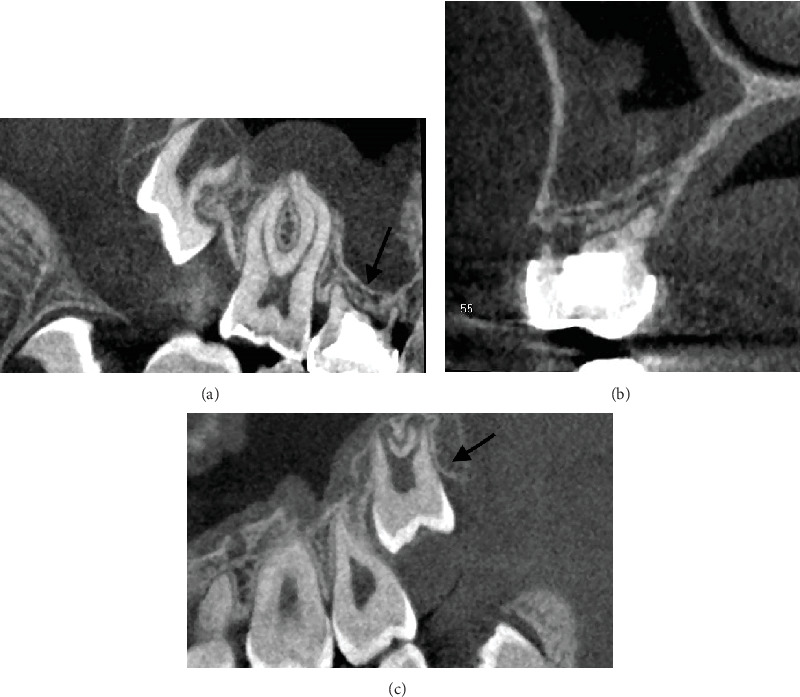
Cone beam tomography was taken to fully understand the condition of the alveolar bone in the recipient site and to evaluate if the third molar was a suitable donor tooth. (a) From the coronal view, the dimensions of the deciduous molar could be measured. (b) The sagittal view revealed how thin the maxillary bone is between the oral cavity and the sinus. (c) The roots of the third molar had developed to three-quarters of their length. The width of the crown exceeded the width of the persisting deciduous tooth in the recipient area.
